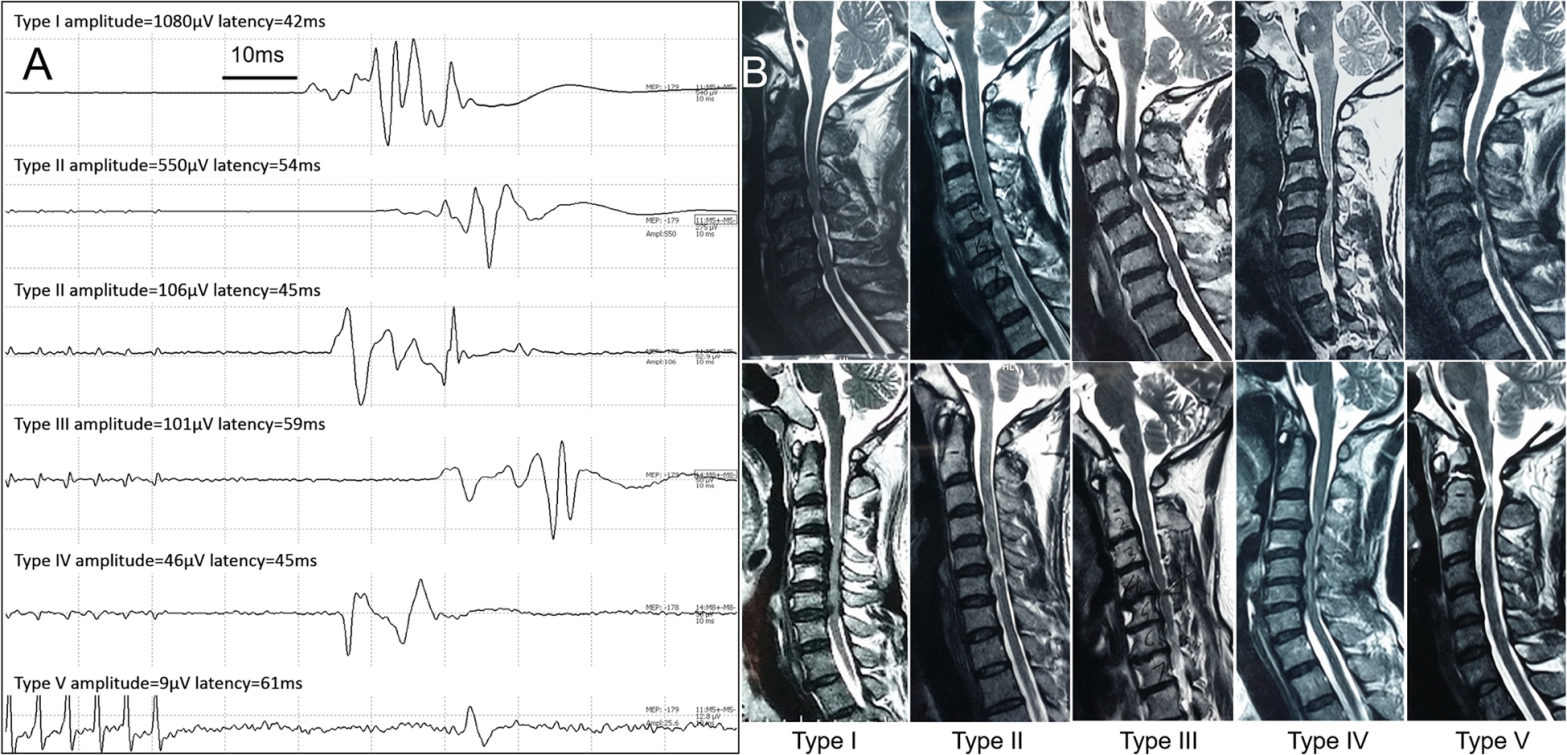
a The representative MEP waveform and parameters among the 5 baseline types. Type II has two subtypes. b The preoperative T2-weighted sagittal magnetic resonance image (MRI) from the patients with different MEP baseline types (Types I, II, III, IV and V)
